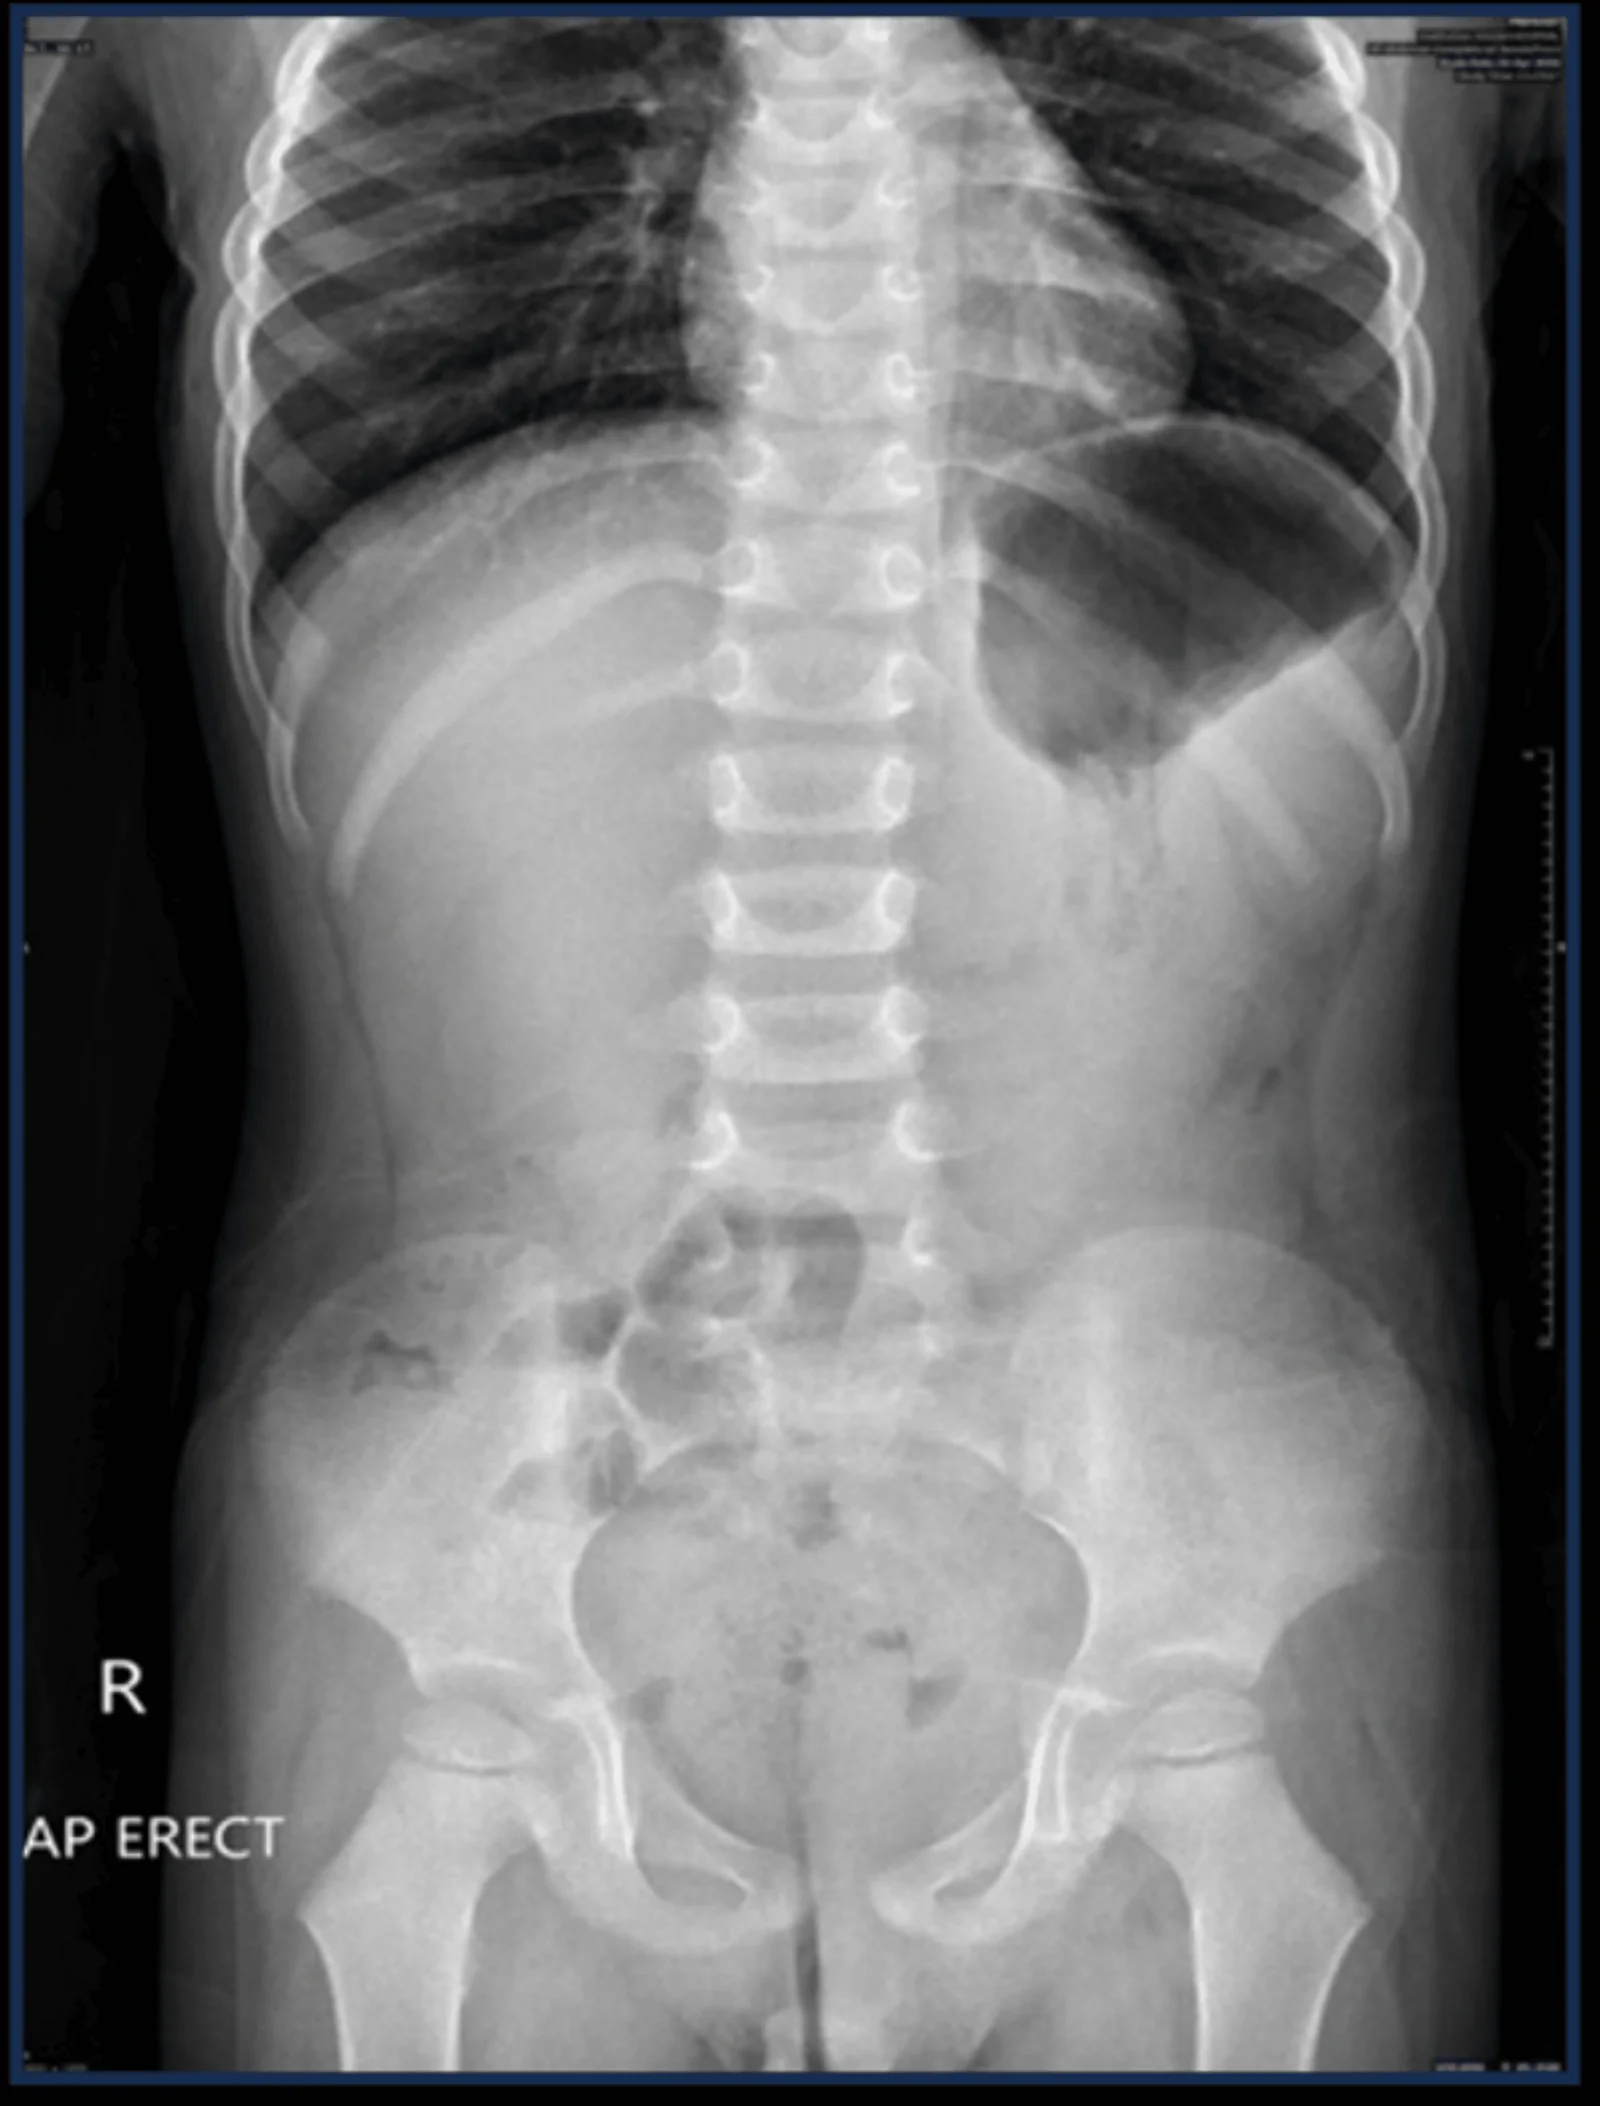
Erect anteroposterior abdominal X-ray demonstrating a normal bowel gas pattern without abnormal air-fluid levels, free intraperitoneal air, or abnormal calcifications
